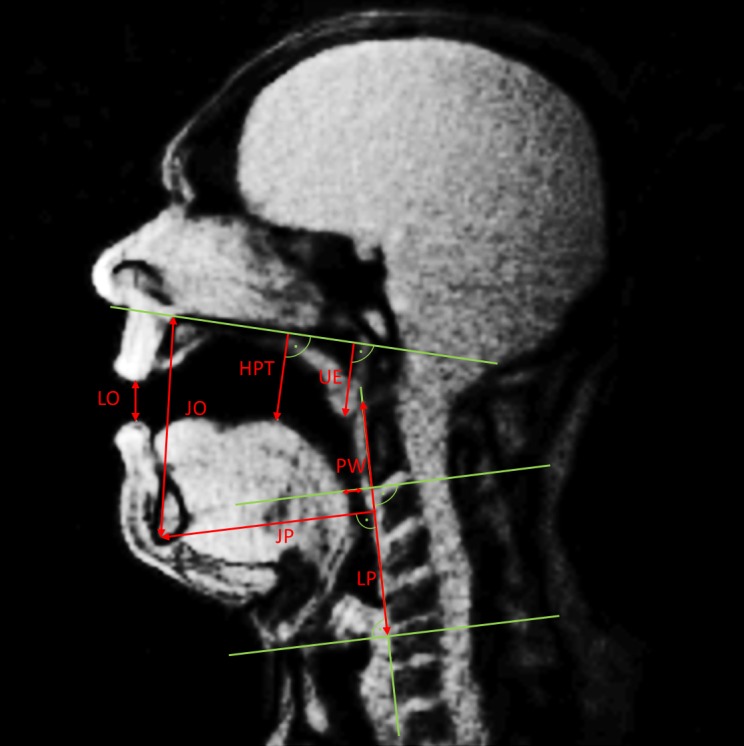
Measured distances in each frame of the MRI material (red arrows), as described previously in detail [8–11,15]. The auxiliary lines are shown in green. LO (Lip Opening), JO (Jaw Opening), JP (Jaw Protrution) HPT (Highest Point Tongue), UE (Uvula Elevation), LP (vertical Larynx Position).
